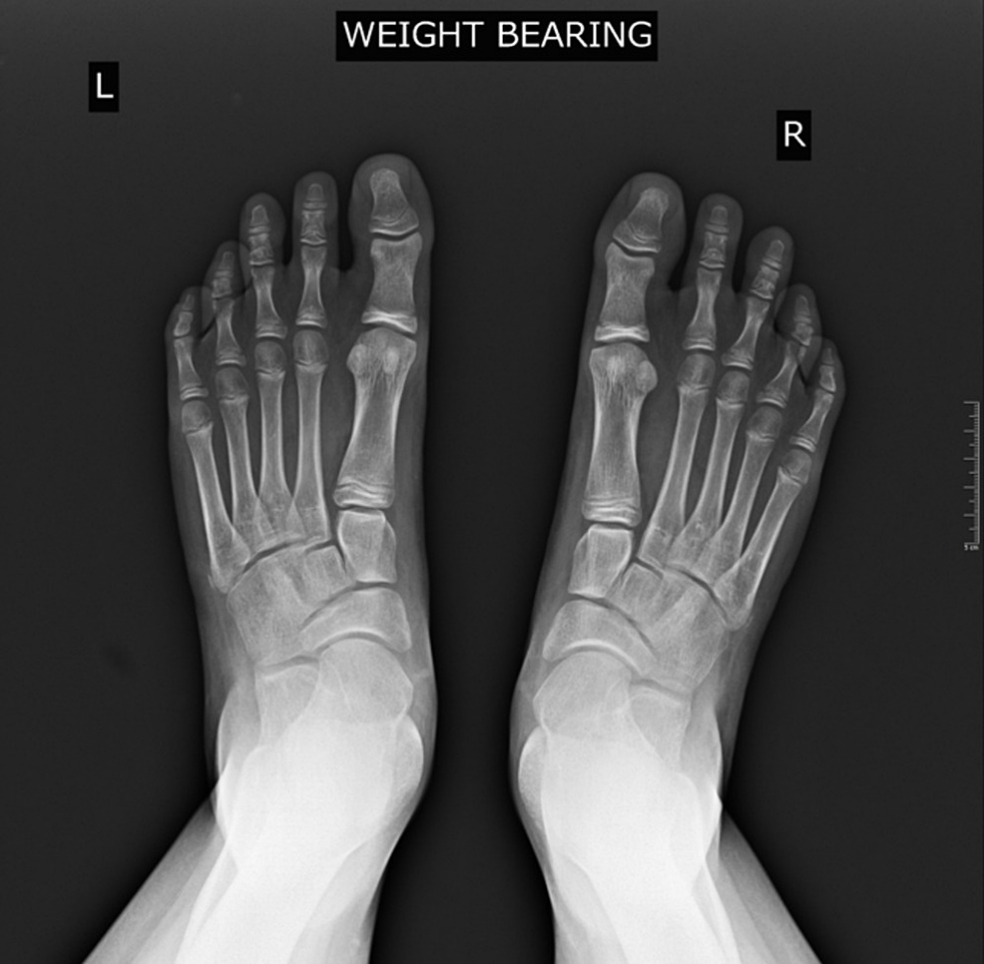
Anteroposterior preoperative bilateral weight-bearing feet X-ray.
